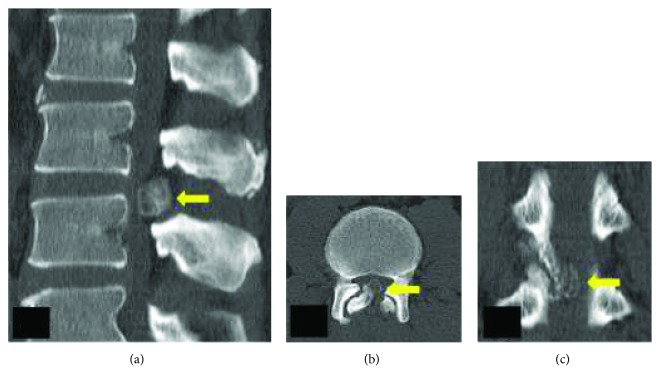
(a) Sagittal CT image shows the huge osseous lesion causing severe compromise of the spinal canal. (b and c) Axial and coronal images demonstrating the attachment to the right L2 inferior articular process.
